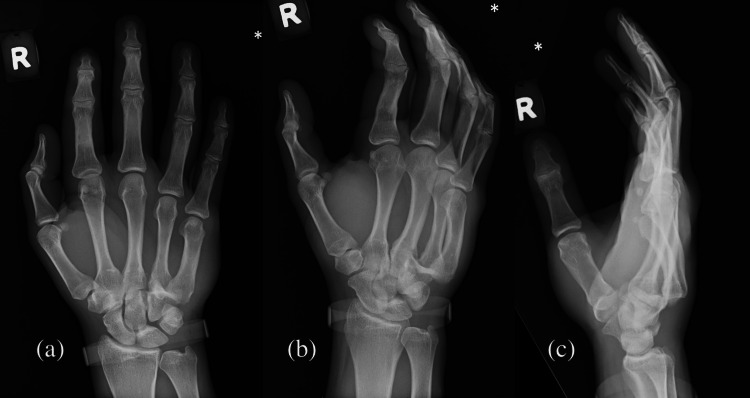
X-ray images showing dorsal dislocation of the ring and little finger carpometacarpal joints. (a) Anteroposterior view, (b) oblique view, and (c) lateral view.
